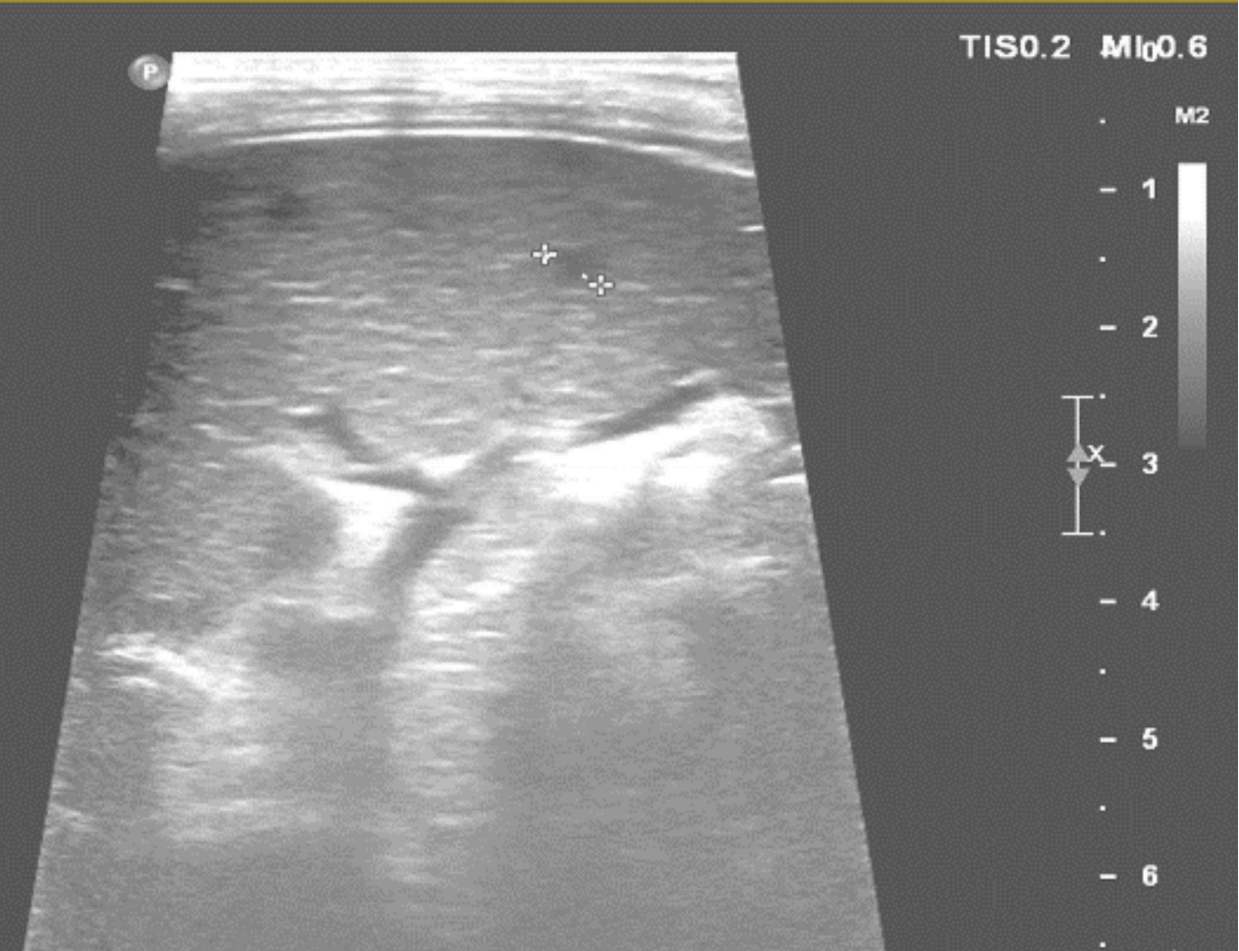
Abdominal ultrasound scan for P1 (4 mo old) showing an enlarged spleen (79-mm diameter) with multiple ill-defined hypoechoic areas (with a maximum size of 4 mm) in the parenchyma.
